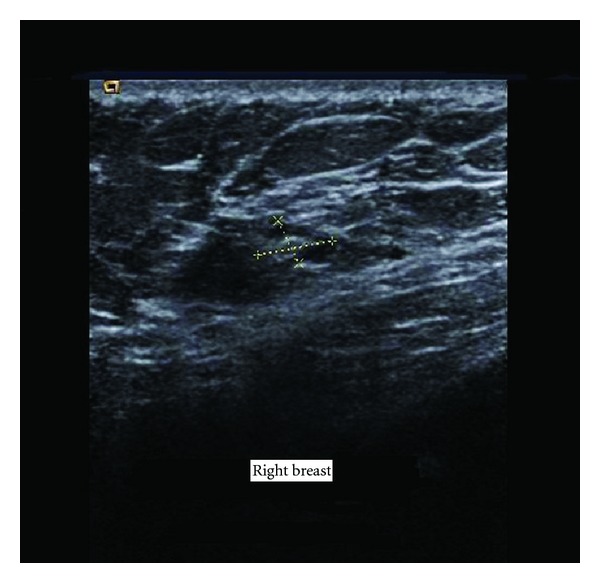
Ultrasonography showed heterogeneous mass with cystic and solid components, measuring 19 × 10 × 11 mm.
